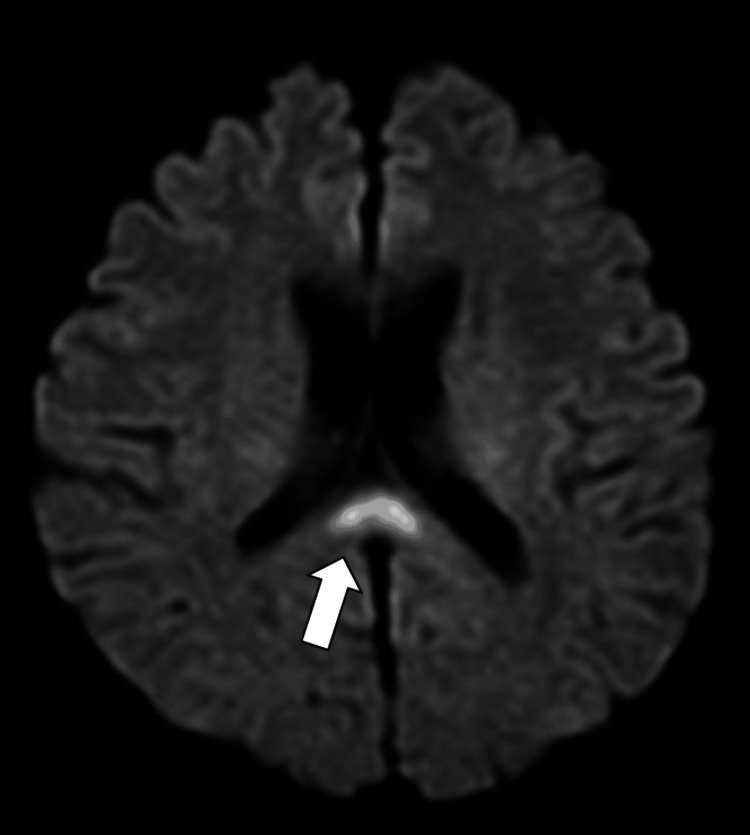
Diffusion-weighted imaging of the head MRI A high signal in the center of the splenium of the corpus callosum (SCC) extending irregularly into the lateral portion of the SCC was revealed (arrow).
